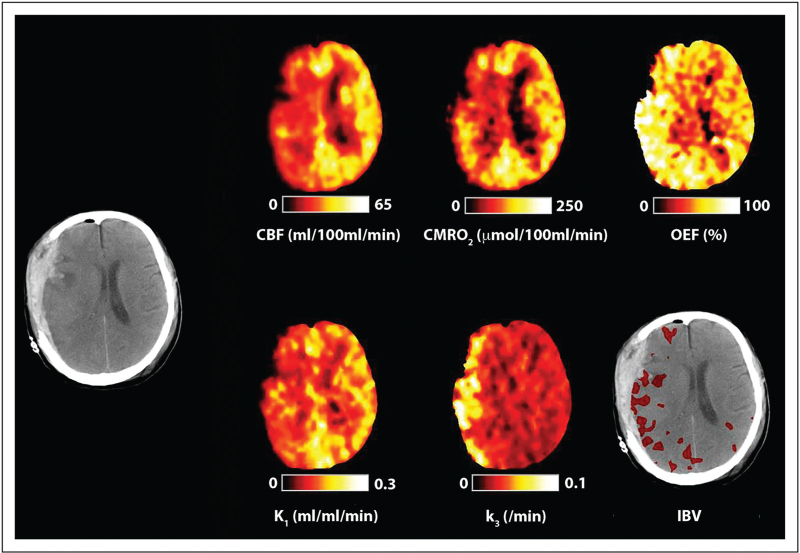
Cerebral ischemia. Computed tomography, cerebral blood flow, cerebral metabolic rate of oxygen, oxygen extraction fraction, ischemic brain volume (IBV) and PET ^18^F-fluorodeoxyglucose kinetic parameters for K_1_ (glucose delivery) and k_3_ (glycolysis) obtained in a 40-year-old female within 24 h of severe traumatic brain injury resulting from a fall. The computed tomography scan was obtained following evacuation of a subdural hematoma and demonstrates residual subdural blood with minimal midline shift and underlying hemorrhagic contusions. Cerebral blood flow, cerebral metabolic rate of oxygen and (K_1_) glucose delivery are reduced, whereas oxygen extraction fraction and k_3_ (glycolysis) are increased within the hemisphere underlying the subdural. These findings are consistent with classical ischemia.
